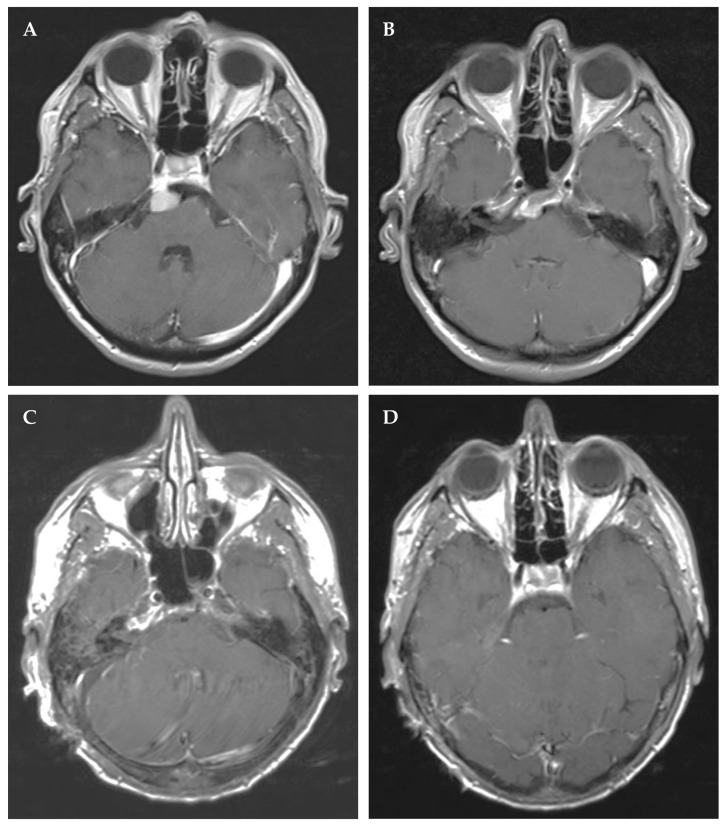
Right petroclival meningioma in a 58-year-old male with tinnitus. The small tumor had been detected 3 years prior to surgery, at an external facility. Preoperative contrast-enhanced T1 magnetic resonance imaging (MRI) in axial (A,B) reconstructions without affection of the brain stem or invasion into the cavernous sinus. Postoperative imaging after complete Simpson I resection via a retrosigmoid approach (C,D) without new postoperative deficits.
